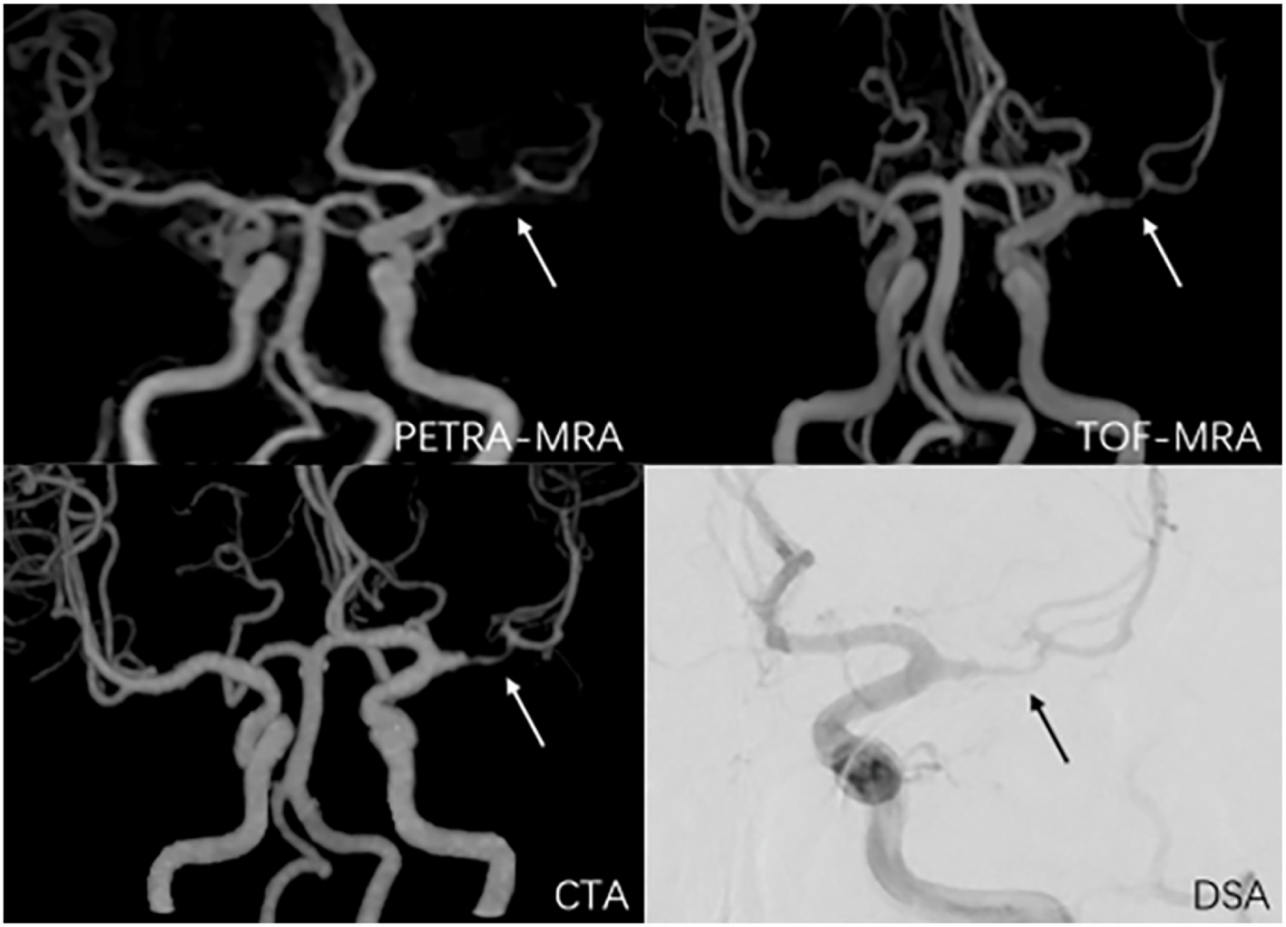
A 38-year-old man with symptomatic left middle artery stenosis. The arrow marks the location of the lesion. The degree of stenosis was 80.9% on PETRA-MRA, 89.1% on TOF-MRA, 88.3% on CTA, and 82.3% on DSA. Lesion length was 6.98 mm on PETRA-MRA, 7.41 mm on TOF-MRA, 7.83 mm on CTA, and 6.5 4 mm on DSA.
